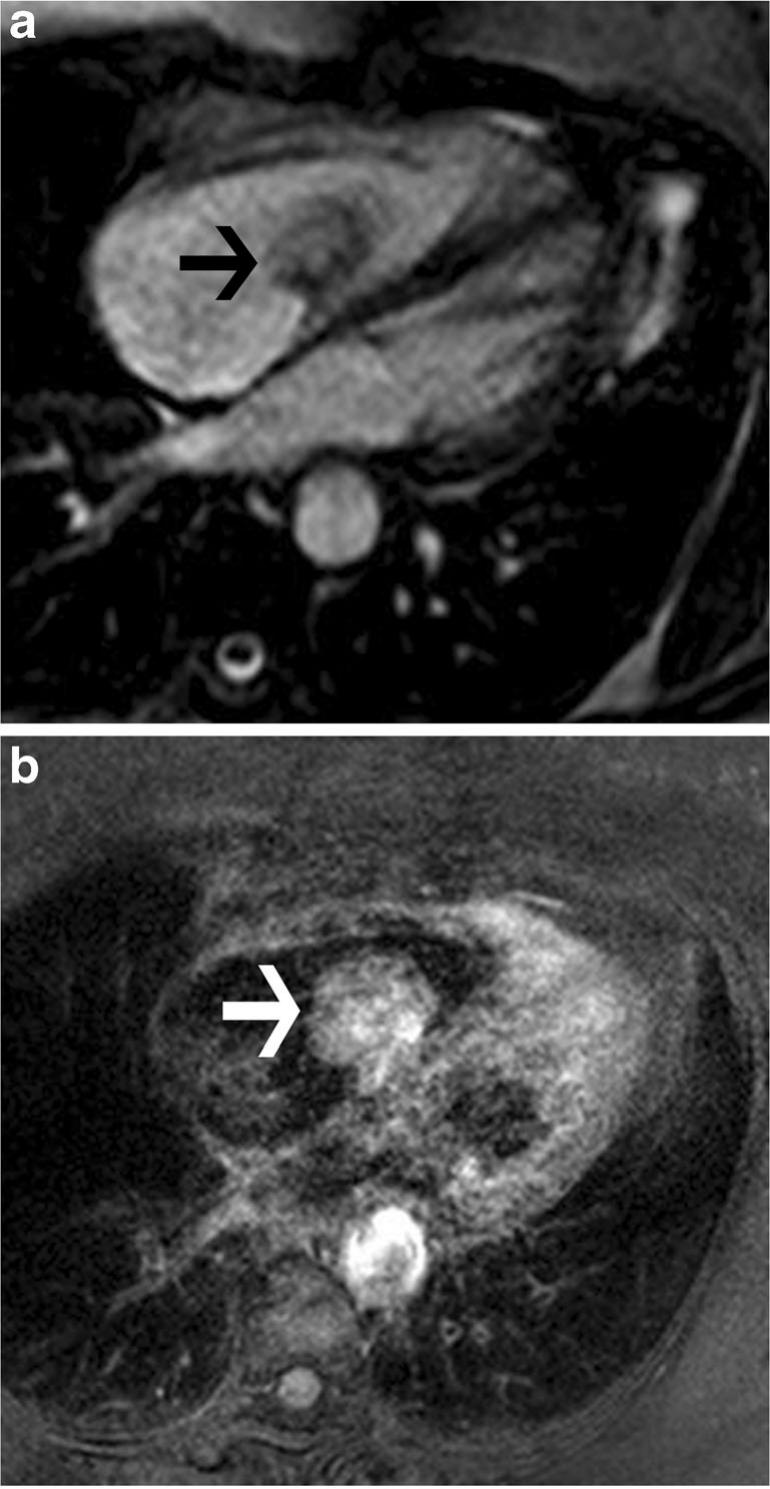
Myxoma. (a) Four-chamber steady-state free precession (SSFP) image shows an iso- to hypointense mass attached to the tricuspid valve. (b) Post-contrast T1-weighted image shows heterogeneous contrast enhancement. This was a biopsy-proven myxoma
